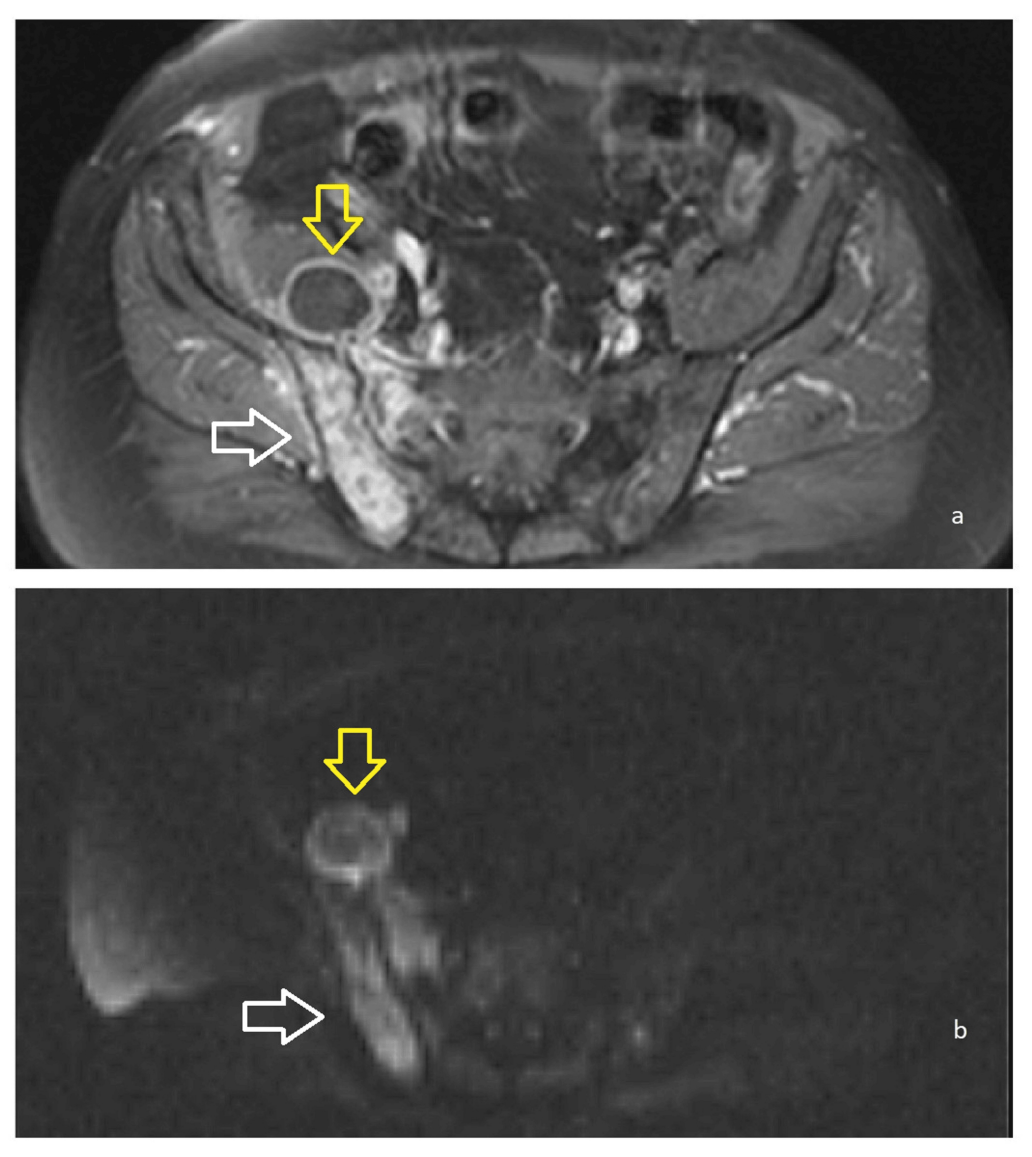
Magnetic resonance imaging (MRI) of the pelvis T1W1 sequence (a) and DWI (diffusion-weighted imaging, b): Depiction of abnormal increased enhancement and restricted diffusion of the right iliac bone, the right wing of the sacral bone, the right gluteus minimus and medius, and the ipsilateral piriformis muscle indicative of osteomyelitis (white arrow). A fluid-containing lesion with peripheral enhancement is imaged at the right iliopsoas muscle (yellow arrow).
